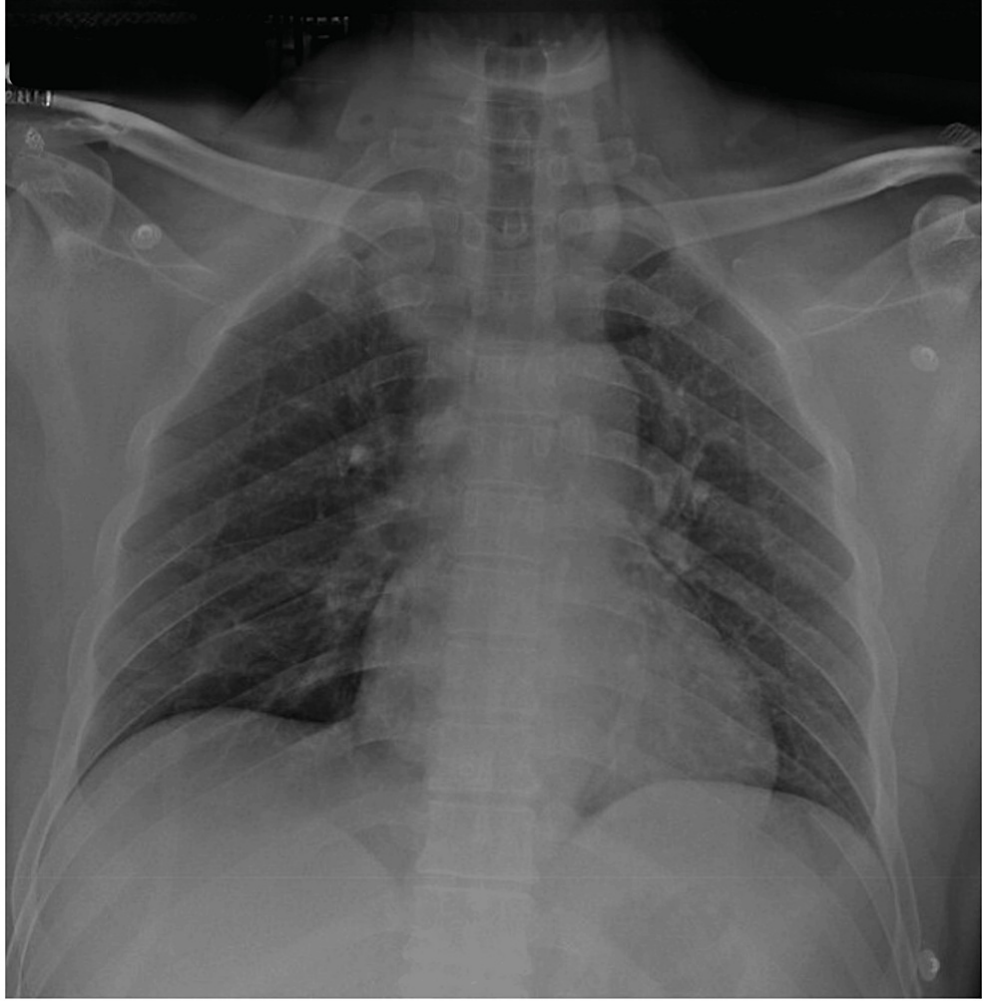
The chest X-ray demonstrated no abnormalities, with no evidence of pneumothorax, pulmonary contusion, or rib fractures.
